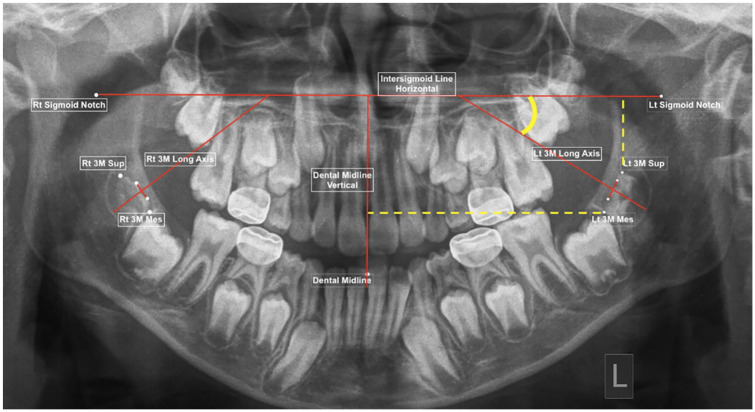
Panoramic radiograph landmarks and lines.
